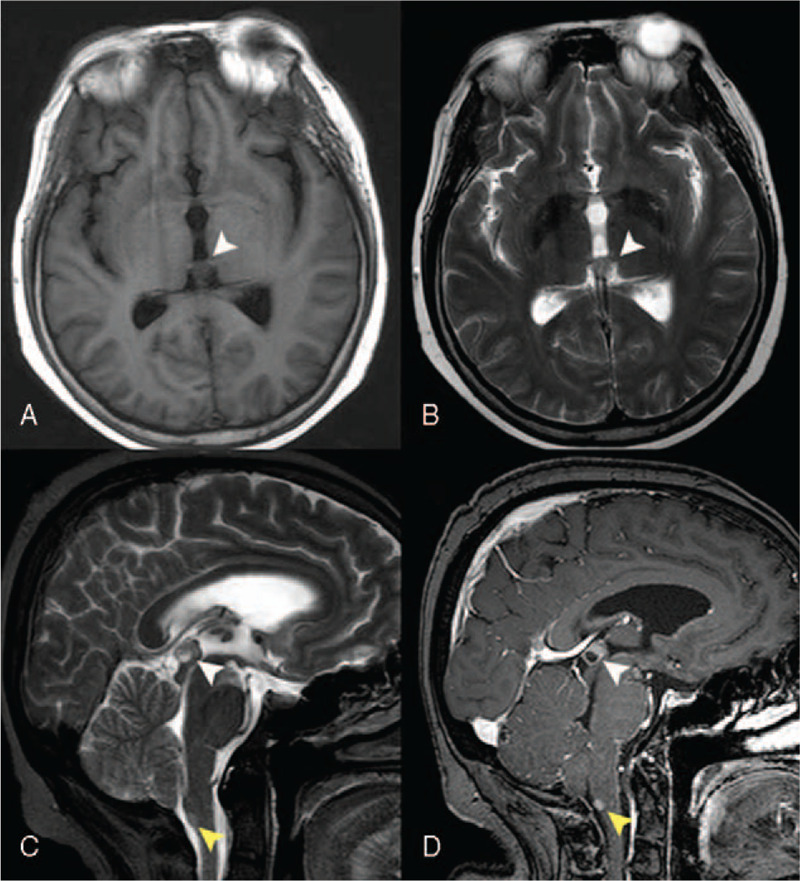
Cranial MRI showed an isointense nodular lesion in the pineal region on T1-weighted (panel A) and T2-weighted phases (panel B), which lead to mild hydrocephalus. Sagittal view of T2-weighted MRI also revealed a hyperintense lesion in the dorsal medulla (panel C, yellow arrowhead). Both two lesions were enhanced after gadolinium enhancement.
